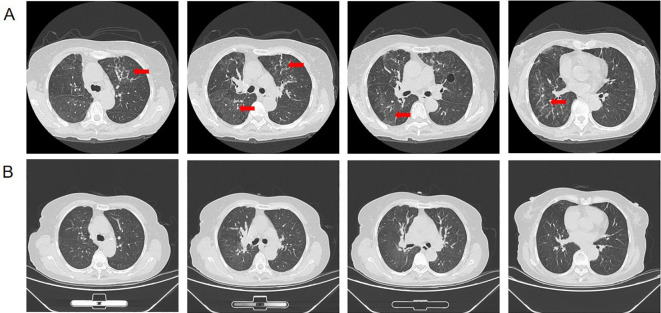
Serial thoracic HRCT before and after management of Osi-ILD. (A) Thoracic HRCT obtained at the onset of symptoms after 3 months of osimertinib therapy shows characteristic bilateral, confluent GGOs with fine reticulation, consistent with Osi-ILD. (B) Follow-up HRCT acquired 8 weeks after osimertinib discontinuation confirms complete radiological resolution of the interstitial lung abnormalities.
